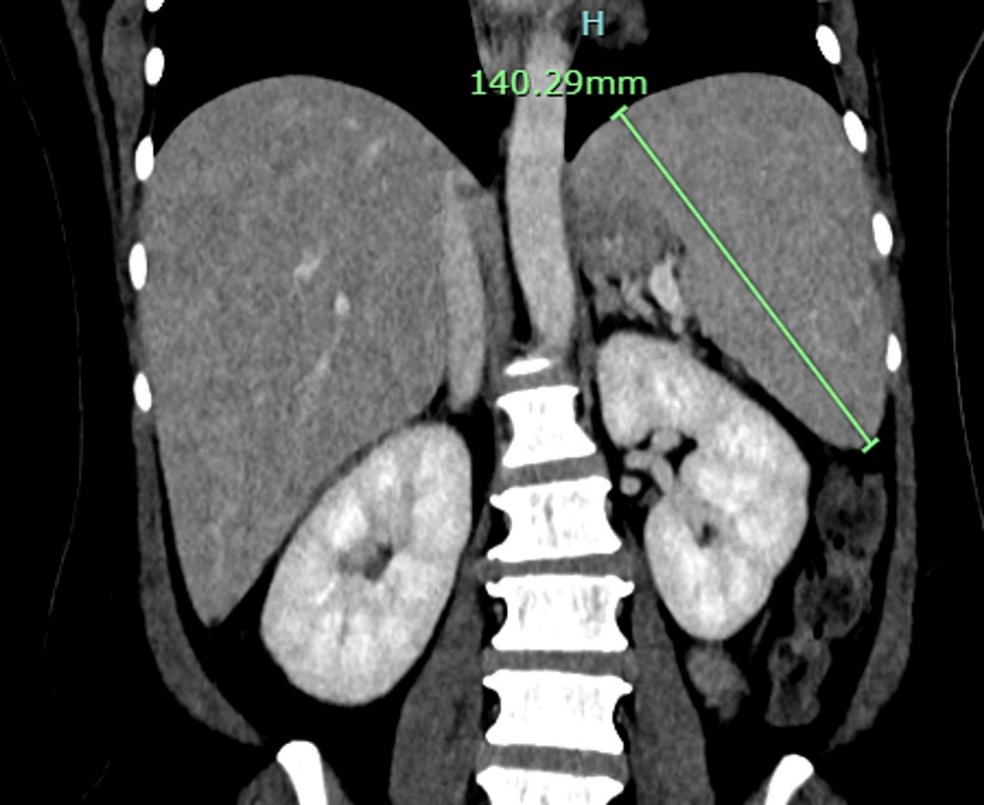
Selected coronal reconstructed image of contrast-enhanced CT abdomen showing mildly enlarged spleen measuring about 14 cm with no focal lesion.
